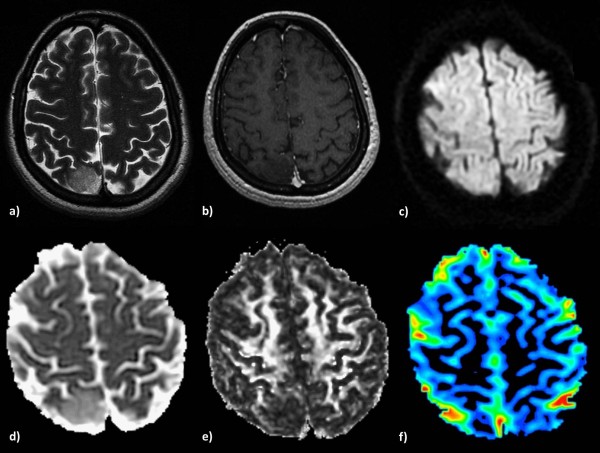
Low-grade glioma in a 55-year-old woman. a) High signal intensity on a T2-weighted image, b) no contrast enhancement on a 3D-SPGR image and c) an isointense signal on a diffusion-weighted image. The lesion shows increased ADC (d), lower FA (e) and no significant perfusion (f) on the corresponding parametric maps.
